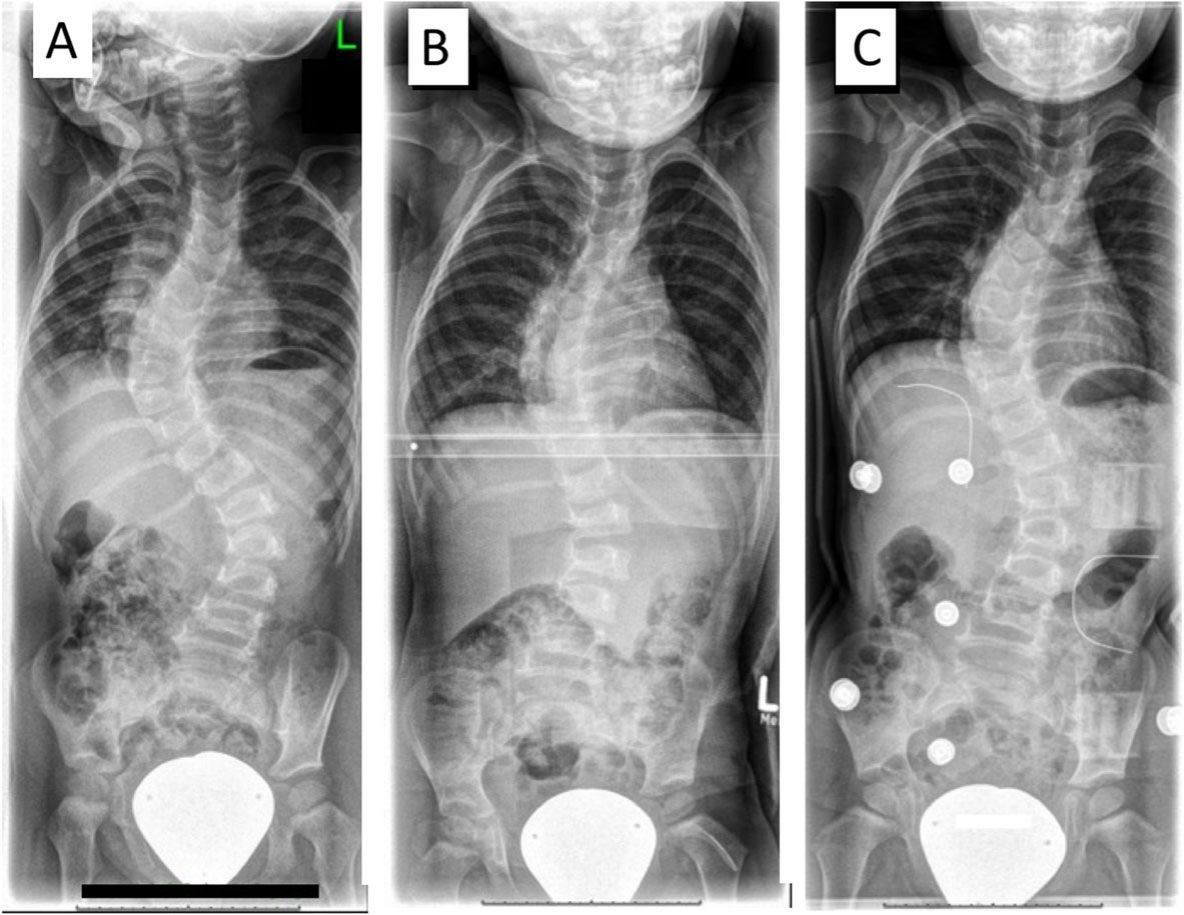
Consecutive radiographs showing the spinal column of the same patient before treatment in the first cast and in brace. a The spinal column before casting, CA = 66°. b Radiograph after implementation of the first Risser cast. The curve was reduced to 42°. c After the third Risser cast the Chêneau brace was applied, the remaining CA = 39° in the brace
